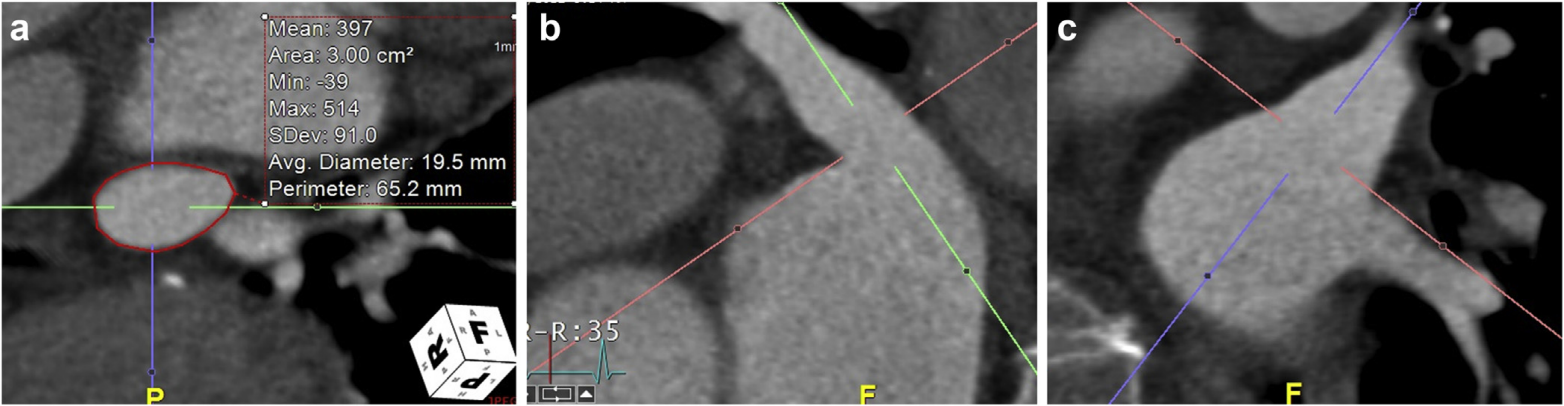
Baseline computed tomography angiography (CTA) measurements of the left atrial appendage (LAA). (a) Axial view; (b) Sagittal view; (c) Coronal view.
